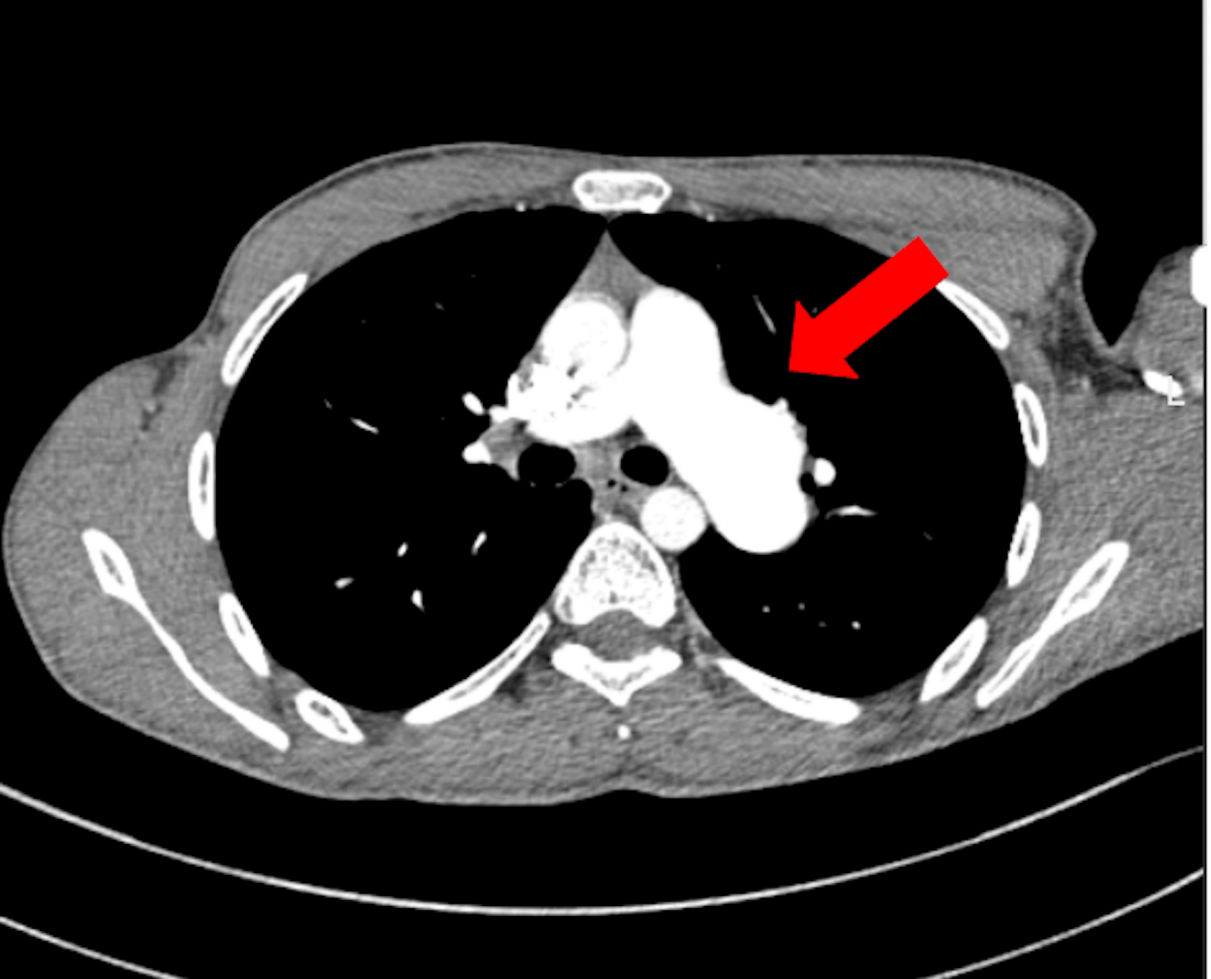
Axial CTPA Showing Left Pulmonary Artery Dilatation Marked dilatation of the left pulmonary artery (red arrow) is seen, consistent with post-stenotic pulmonary artery dilatation in the setting of severe pulmonary valve stenosis. No intraluminal filling defects suggestive of pulmonary embolism are observed. CTPA: computed tomography pulmonary angiography
